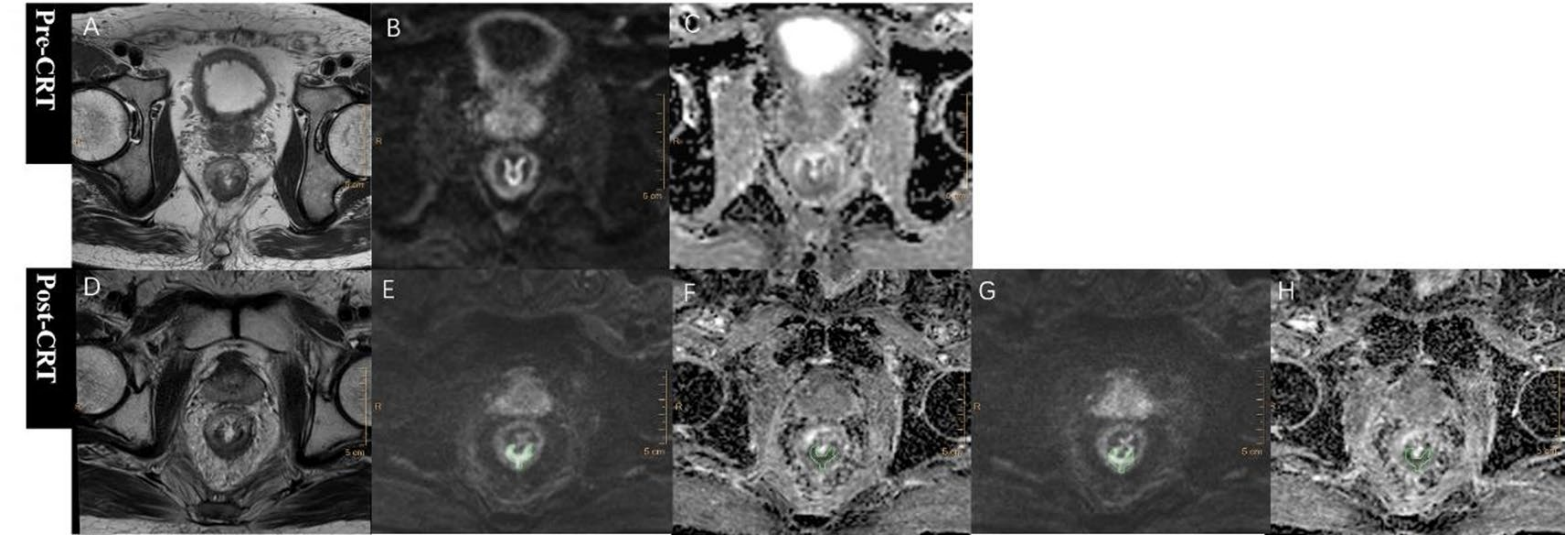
Example of RESOLVE, SMS-RESOLVE DWI and corresponding ADC maps of a 66-year-old male patient, who was diagnosed ypT3N0 and TRG2 after surgery. A T2-weighted image before neoadjuvant therapy. B RESOLVE DWI image with b value of 1000s/mm^2^ with ROI delineation of residual tumor. C Corresponding ADC map of RESOLVE DWI, and the residual tumor showed ADC value of 1.089*10^− 3^mm^2^/s. D T2-weighted image after completion of neoadjuvant therapy. E SMS-RESOLVE DWI image with b value of 1000s/mm^2^ with copied ROI delineation of residual tumor from RESOLVE DWI. F Corresponding ADC map of SMS-RESOLVE DWI, and the residual tumor showed ADC value of 1.035*10^− 3^mm^2^/s. For clearer visualization of the underlying image anatomy, please see the same cases without ROIs in Supplementary Fig. 1.
